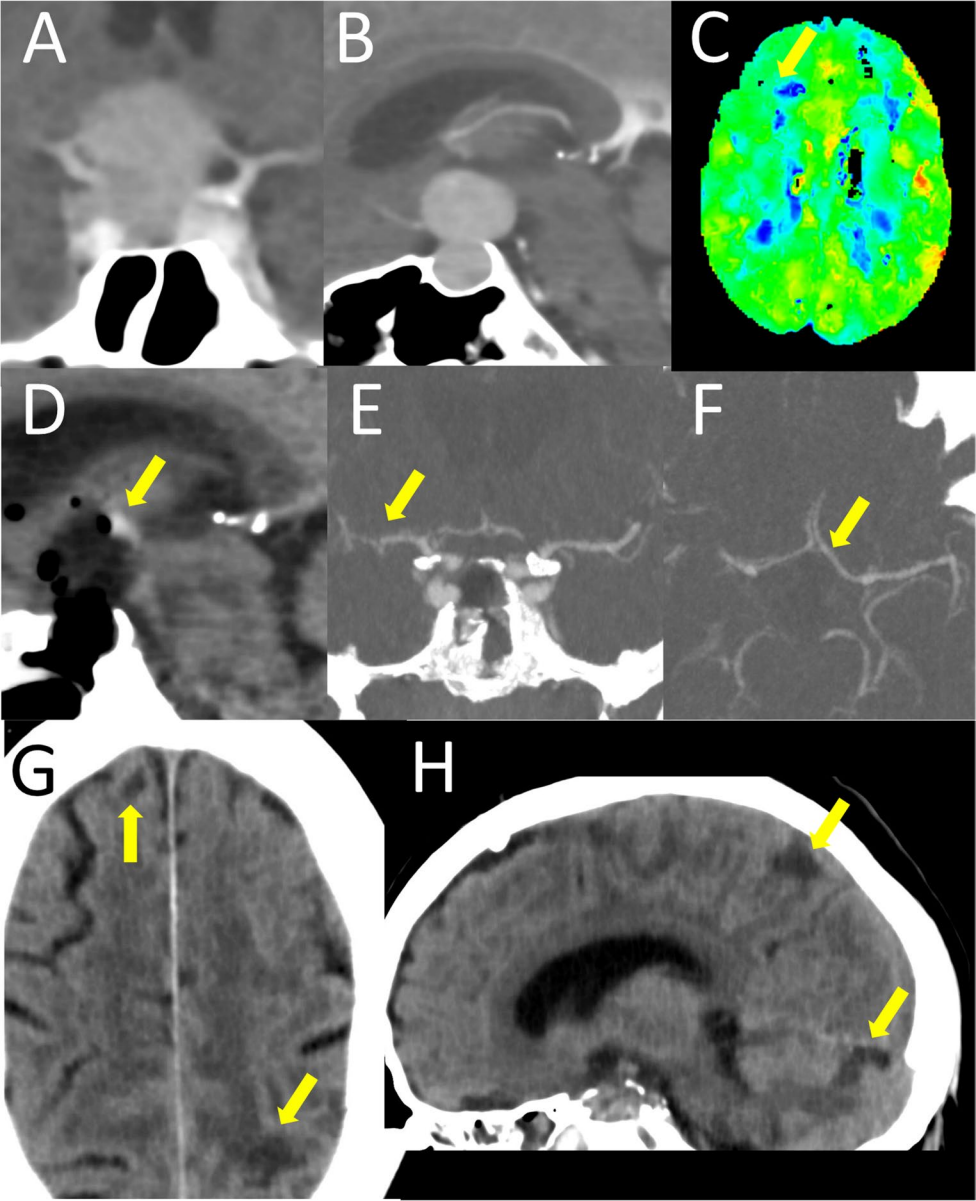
Case 2–54 year old male with non-functioning pituitary neuroendocrine tumor. A (coronal) & B (sagittal): contrast-enhanced cranial CT imaging showing a sellar lesion with suprasellar extension and snowman-like configuration with contact to both A1 segments of the anterior cerebral arteries. C: Postoperative day 5, Tmax map of perfusion CT, blue color indicates delayed perfusion whereas green, yellow and red areas indicate rapid perfusion, arrow pointing out delayed perfusion in the watershed zone of the anterior and middle cerebral artery. D: Postoperative representative sagittal CT section with arrow pointing out focal postoperative tumor bed hemorrhage. E (coronal) & F (sagittal): contrast-enhanced CT angiography on postoperative day 5 with arrows indicating reduced caliber of both A1 segments of the anterior cerebral arteries and right M1 segment of the middle cerebral artery. G (axial) & H (sagittal): cranial CT without contrast agent, arrows demonstrating lacunar ischemic defects in the watershed area of the anterior cerebral artery as well as a single cerebellar defect
